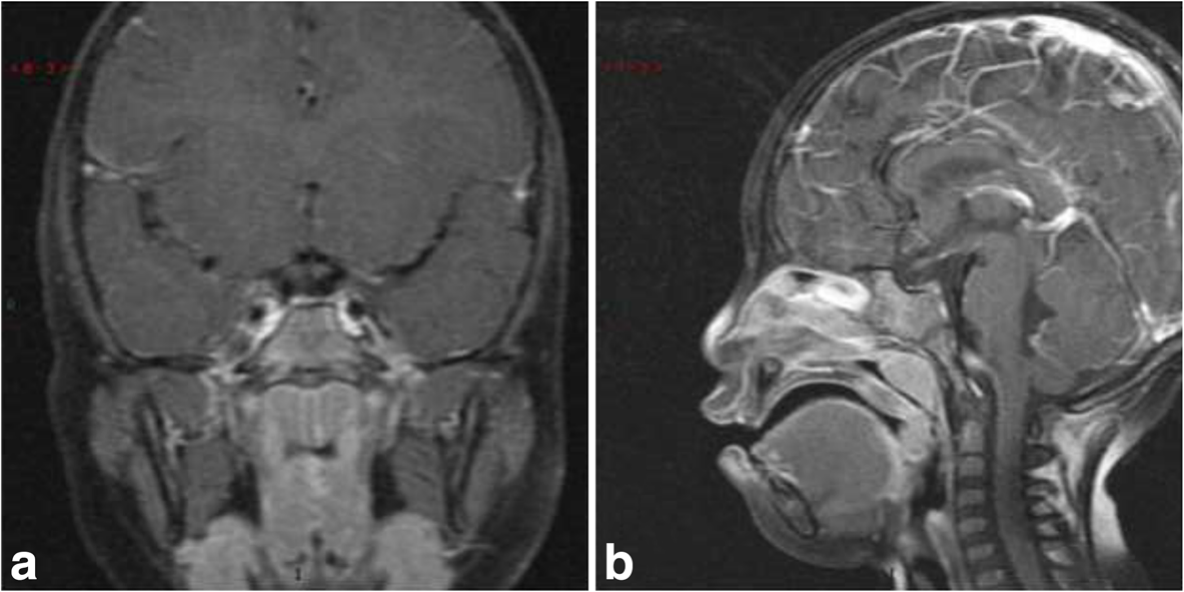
Pituitary gland on MRI. The MRI image results revealed that the Pituitary height was 1.0 mm, much smaller than the normal size, and the neurohypophysis was not seen clearly, which indicated pituitary dysplasia. a Coronal MRI scan of Pituitary Gland. b Sagittal MRI scan of Pituitary Gland
